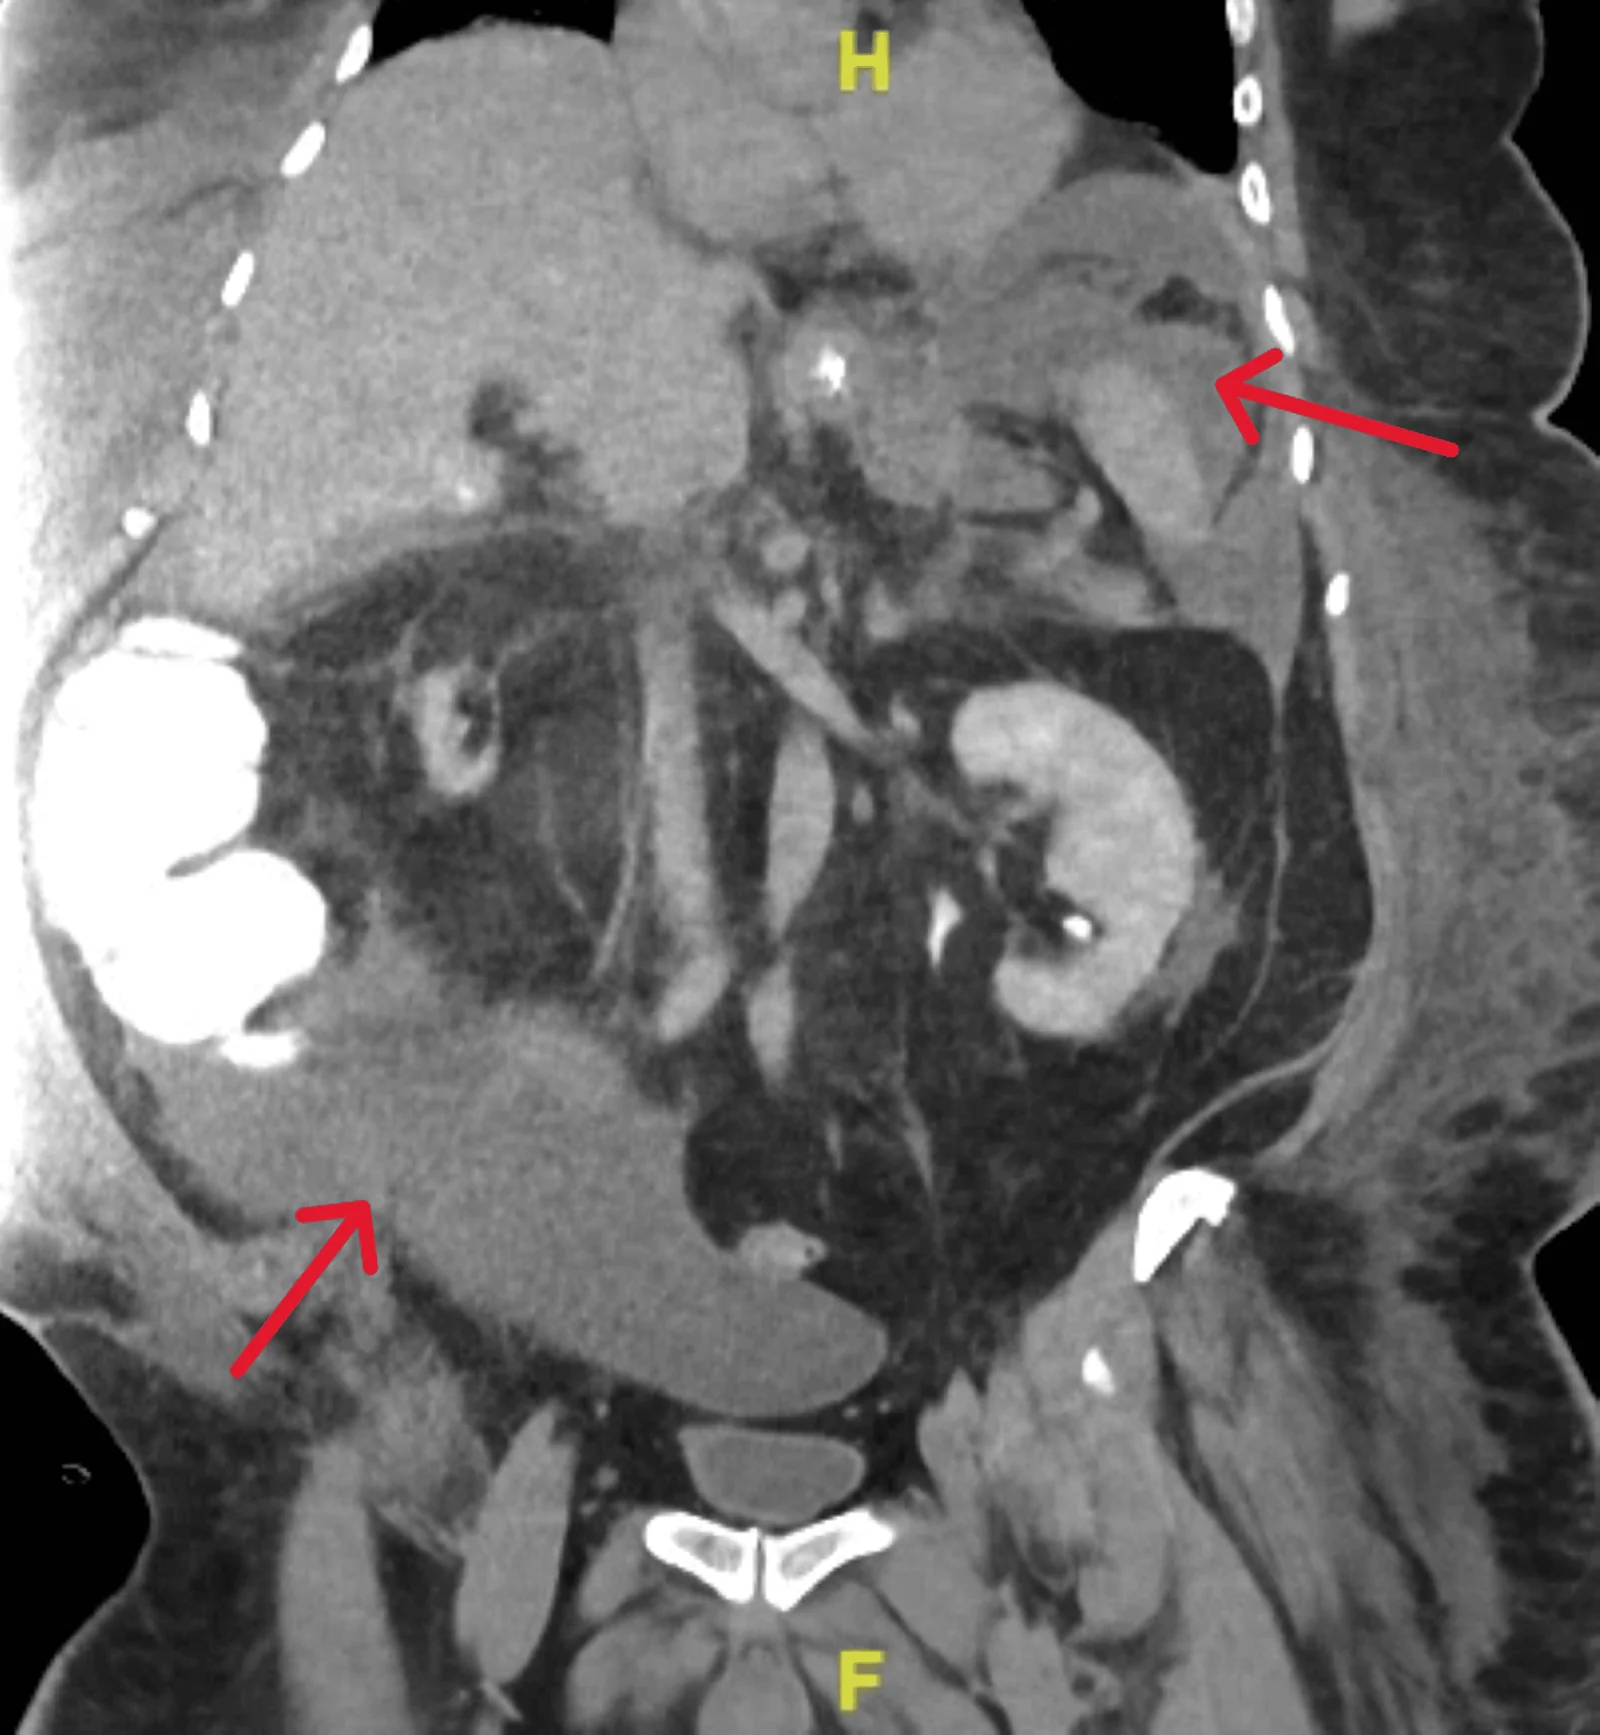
Coronal view of the CT demonstrated intra-abdominal fluid collections (red arrows)
